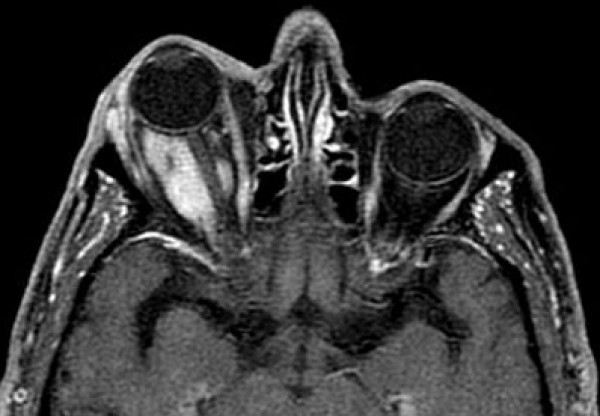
Axial MR image (T1 with fat saturation) after intravenous administration of paramagnetic media. There is homogeneous intense enhancement producing a "tram track" appearance around the hypointense optic nerve. Surrounding structures remain intact.
